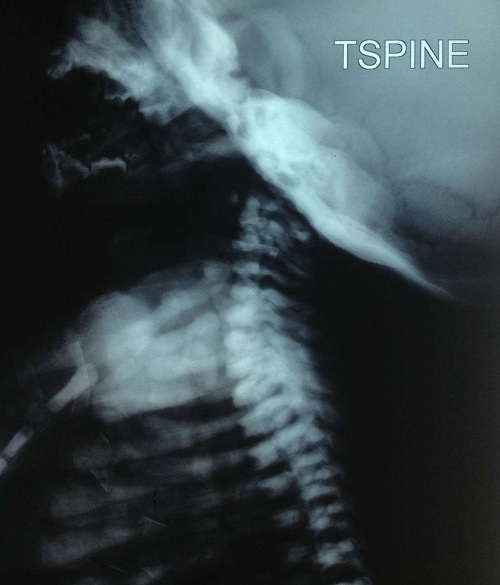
Lateral skull radiograph. Note increased thickness of skull base
